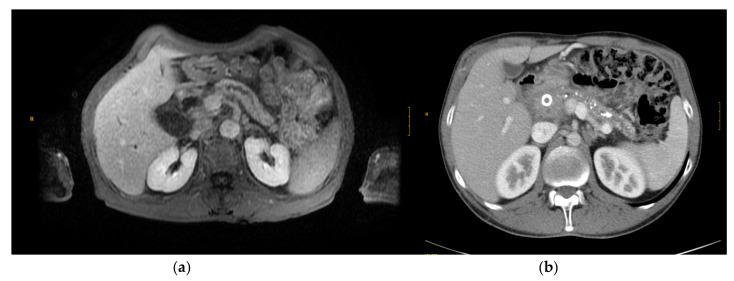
Radiological features of chronic pancreatitis. (a) MR image shows irregular pancreatic duct (PD) contour and ductal dilatation. (b) CT image also shows moderately to markedly irregular PD contour and ductal dilatation. Calcifications in neck and body of pancreas and a metallic biliary stent in head can be observed.
